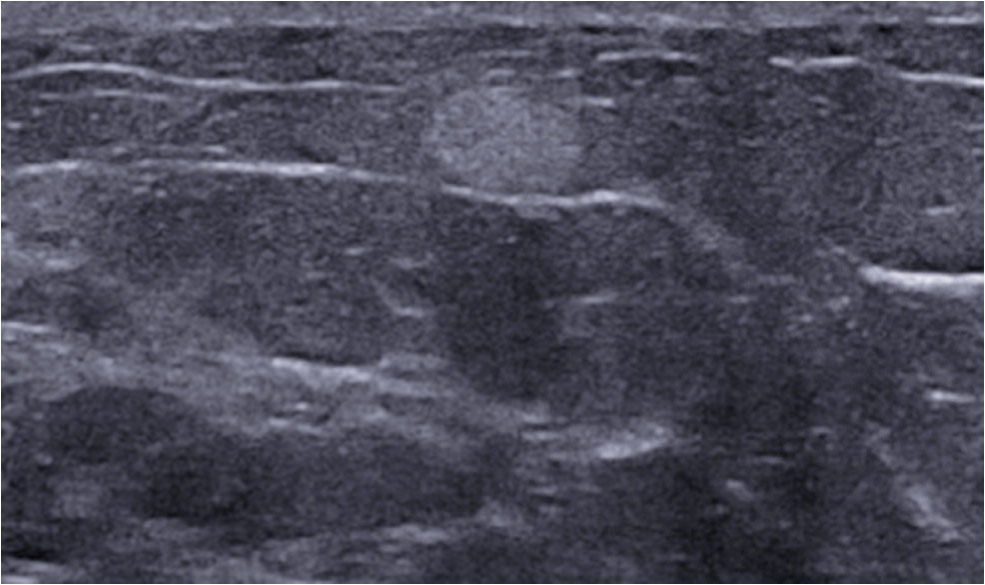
Spindle cell lipoma. Transverse ultrasound scan showing a homogeneous, hyperechoic, round mass with well circumscribed margins
